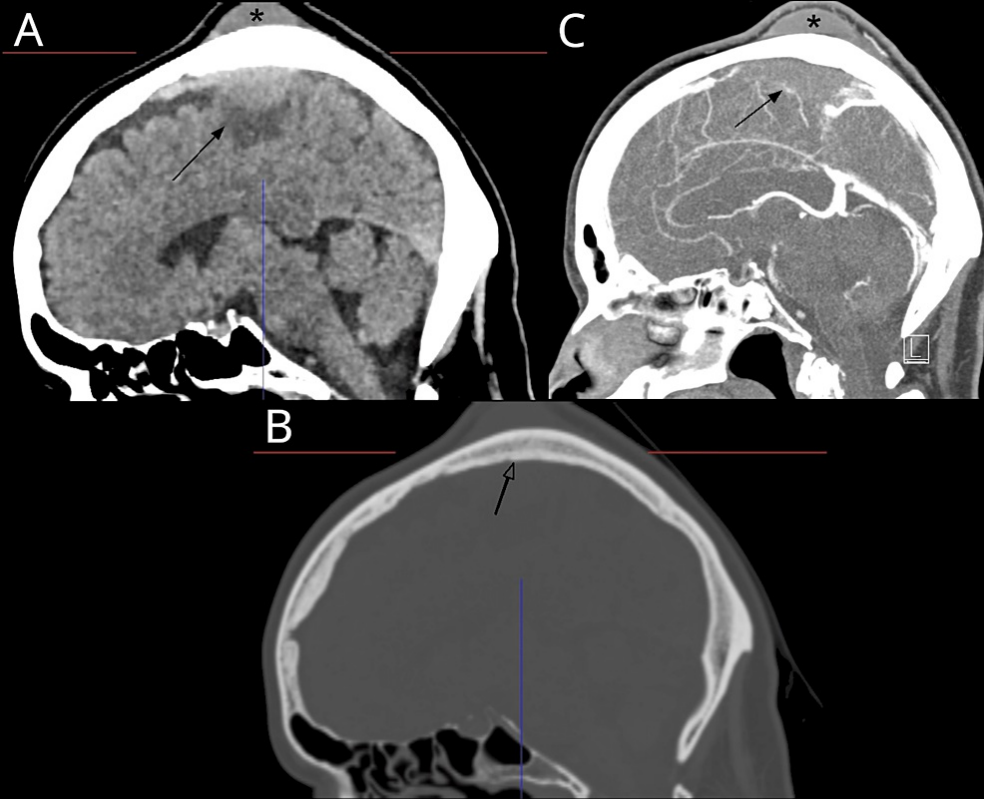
Cranial CT scan. Sagittal CT scan of the head demonstrating an extra- and intracranial mass (asterisk and arrow respectively) (A), sagittal CT head bone window without signs of invasion by the tumor (B), and venous CT angiography showing an obliteration of the superior sagittal sinus due to the intracranial tumor (arrow) (C).
